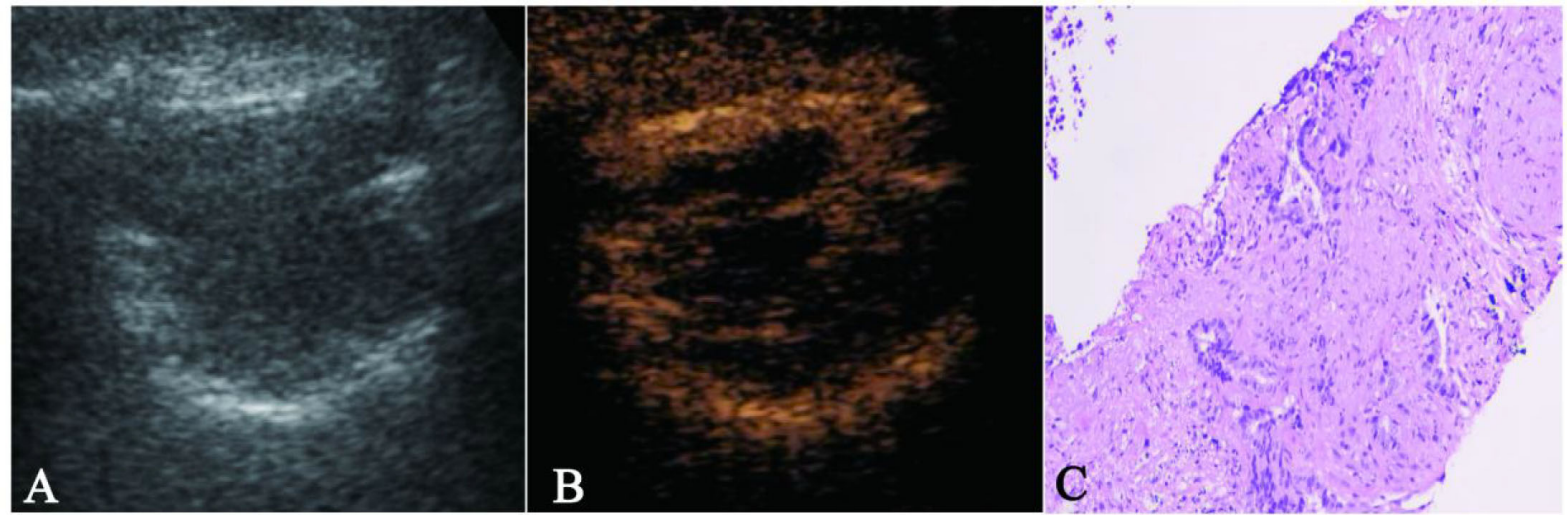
The figure 4A–4C showed a case of 70-year-old male NSCLC with EGFR-wild type. (A) The gray-scale ultrasound shown a hypo-echoic lesion with poor defined boundary. (B) On the contrast-enhanced ultrasound, the lesion exhibited hypo-enhancement with multiple necrotic focis. (C) The pathological image confirmed the lesion as a NSCLC.
